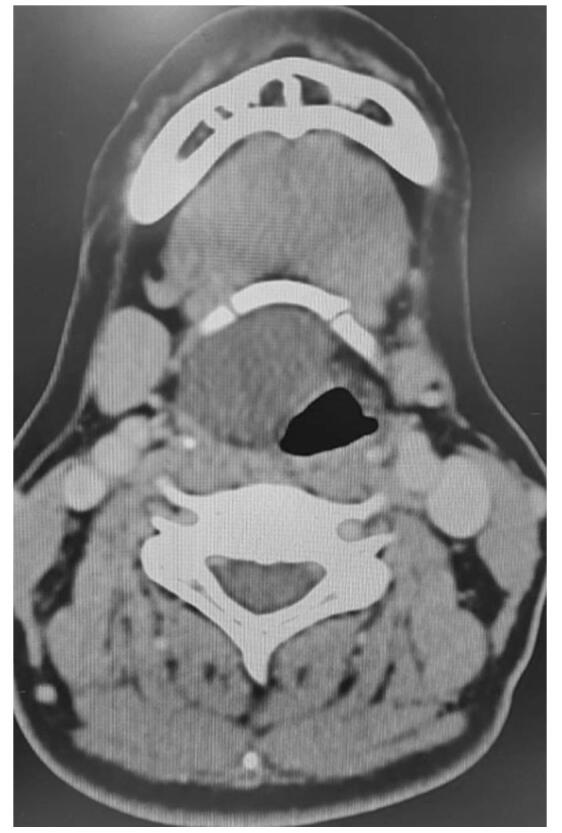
Axial view of neck CT scan: 3.4 × 2.5 × 3.2 cm well-defined hypodense poorly-enhanced nodule at right epiglottis effaced vallecula.
